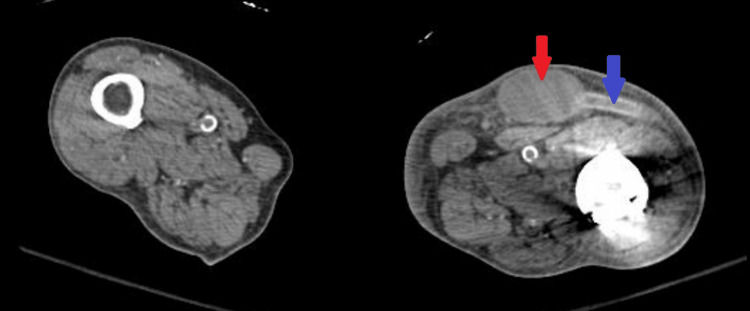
Axial computed tomography angiogram through the left proximal thigh demonstrating a moderate-sized intermediate-density fluid collection surrounding the left lower extremity arteriovenous graft, corresponding to the area of pain and erythema on physical examination Red arrow: fluid collection, blue arrow: arteriovenous graft
